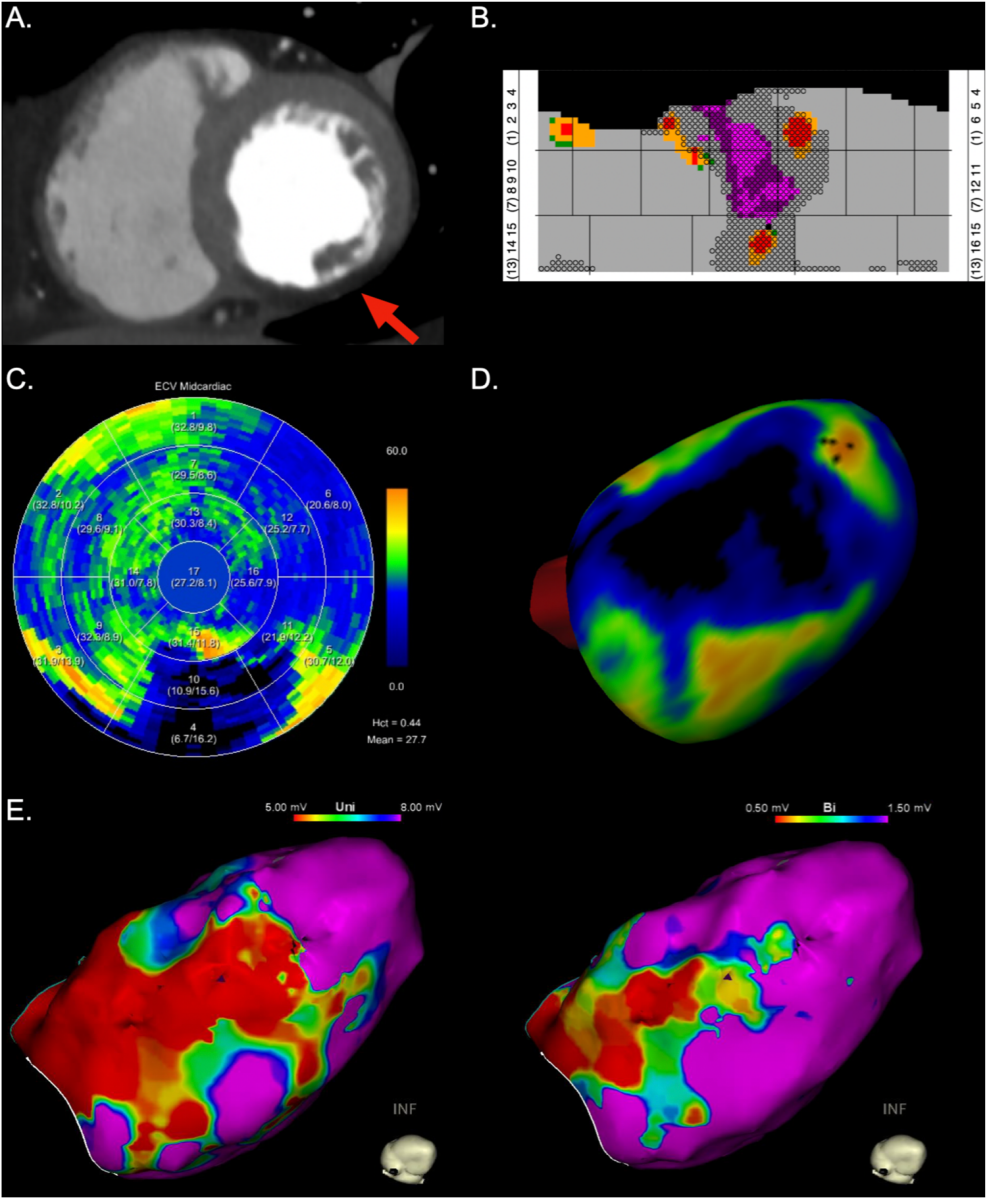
Representative images of the left ventricle of an 82-year-old male patient with ischemic cardiomyopathy. Coronary CT angiography shows myocardial thinning with fatty metaplasia of the inferior wall of the left ventricle (red arrow, A), which is visualized on the atlas map (pink color indicating fatty metaplasia and circles indicating myocardial thinning, B). Adjacent to myocardial thinning, fibrosis reflected by elevated extracellular volume (ECV) can be observed (B–D). Endocardial electroanatomic mapping (EAM) (E) shows corresponding myocardial low-voltage areas of the septal/inferior/inferolateral basal, the inferseptal/inferior/inferolateral midventricular and inferior apical segments in unipolar (< 5.0 mV) EAM and the septal/inferior basal and inferior midventricular segments in bipolar (< 0.5 mV) EAM
